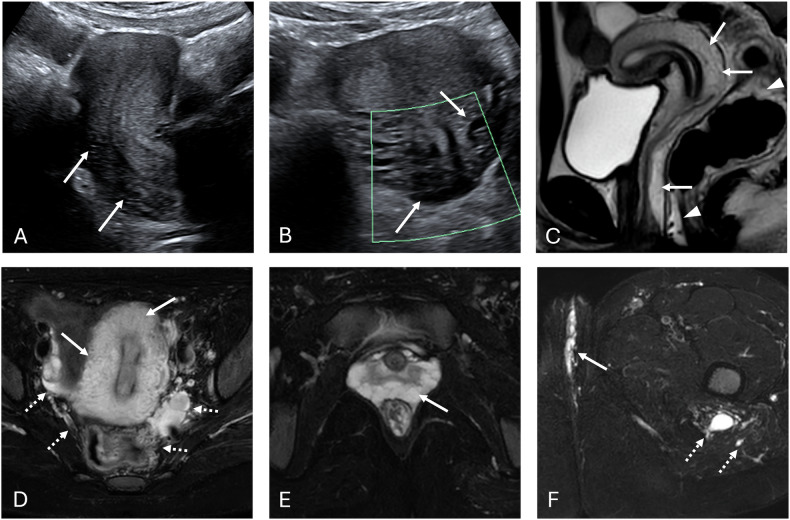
Extensive venous malformations involving the female genital tract in a 13-year-old patient with PROS syndrome and a PIK3CA mutation, treated by alpelisib. A, B Suprapubic ultrasonography images show hypoechoic lacunae within the myometrium and cervix (arrows), with no Doppler signal. C Sagittal T2-W MR images show diffuse T2-hyperintense thickening of the uterine corpus, cervix and posterior vaginal wall (arrows), with a sponge-like pattern, better appreciated than on suprapubic ultrasonography. Note the similar involvement of the rectum and anal canal, indicative of a multi-organ disease (arrowheads). D At the uterine level, E at the vaginal level and F at the perineal and left thigh level, axial T2-W fat-suppressed MR images show diffuse T2-hyperintense thickening of the uterine body, vaginal walls and vulva (arrows). Note the similar involvement of the adjacent pelvic tissues and lower limb, with serpiginous dilated venous structures (dotted arrows) and no visible flow-voids
